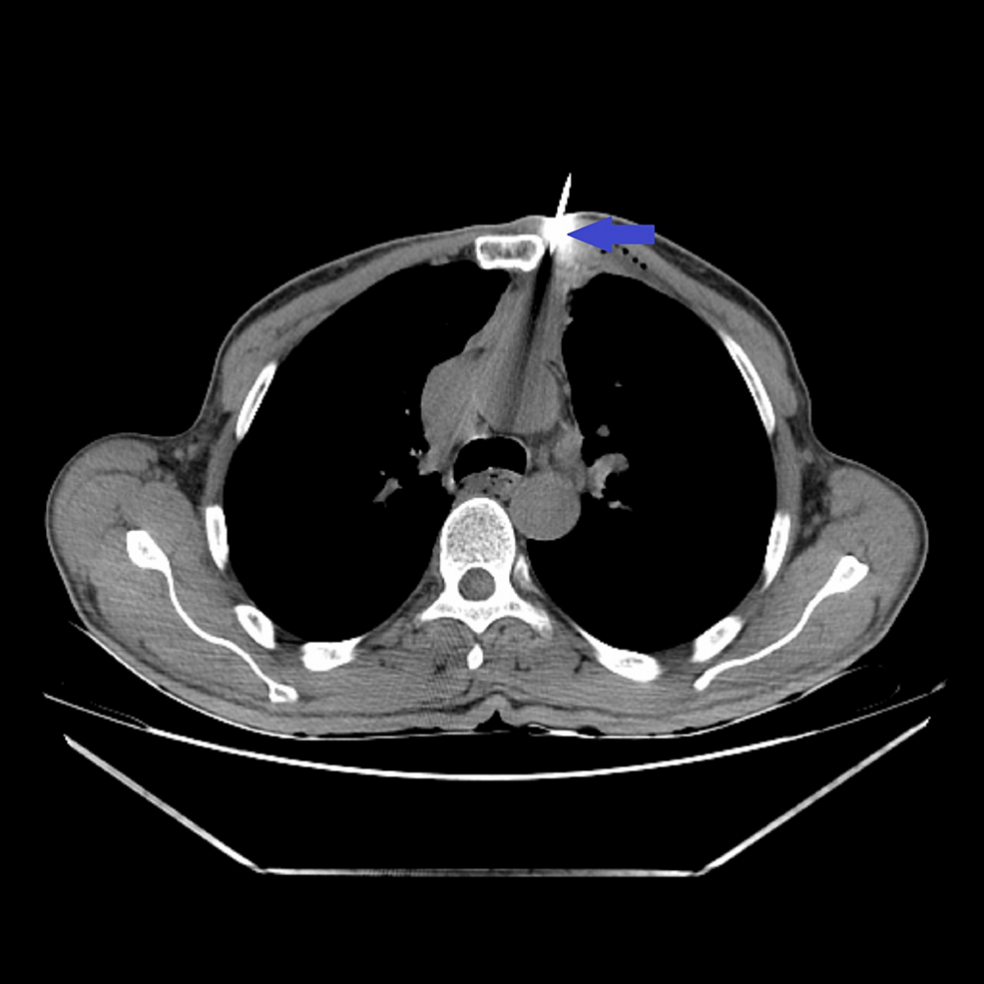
Needle being advanced into the tumor under CT guidance to perform the core biopsy (blue arrow).
